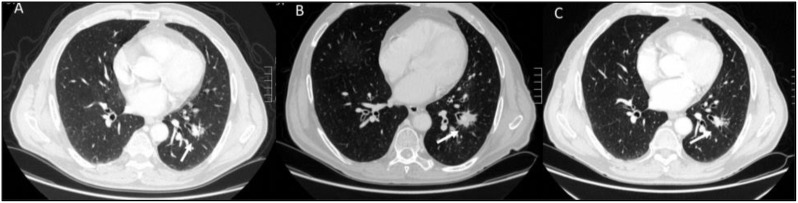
CT imaging evaluation of pseudoprogression and treatment response in a patient with lung cancer undergoing immunotherapy. Axial CT scans: (A) (Baseline CT, Pre-Treatment): The initial CT scan reveals a well-defined, solid lung mass in the left lower lobe. The lesion has irregular margins and is associated with some surrounding ground-glass opacities. (B) (First Evaluation, 3 Weeks After Immunotherapy): Follow-up imaging shows an apparent increase in lesion size (white arrow) with new areas of ground-glass opacity and perilesional infiltration. This pattern is consistent with pseudoprogression. (C) (Second Evaluation, 6 Weeks After Immunotherapy): A subsequent CT scan at 6 weeks demonstrates partial response to treatment, with a reduction in lesion size (white arrow). This confirms that the initial apparent progression was due to pseudoprogression rather than actual tumor growth. From Vanzulli and Albano, (42).
